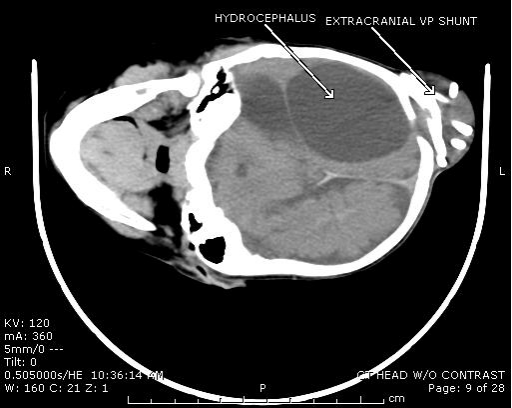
Cross Section CT Showing Coiled Shunt and Hydrocephalus.
